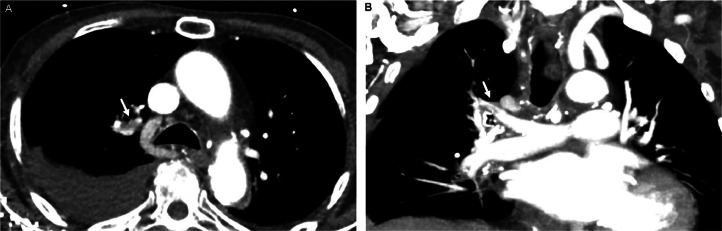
CTA chest Axial (A) and Coronal (B) images demonstrating small right sided pleural effusion and markedly decreased pulmonary embolism in right upper lobe pulmonary arteries (arrow).
